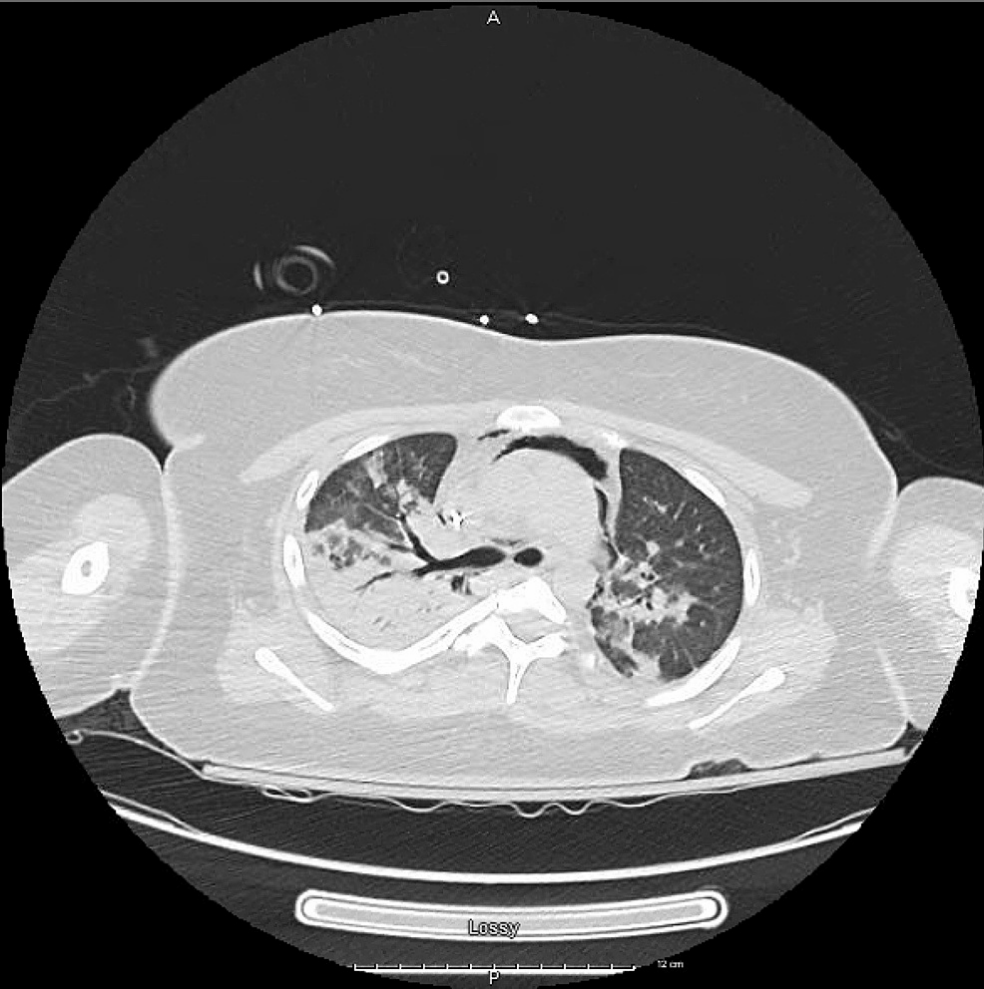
CT scan of the lungs showing bibasilar consolidation
